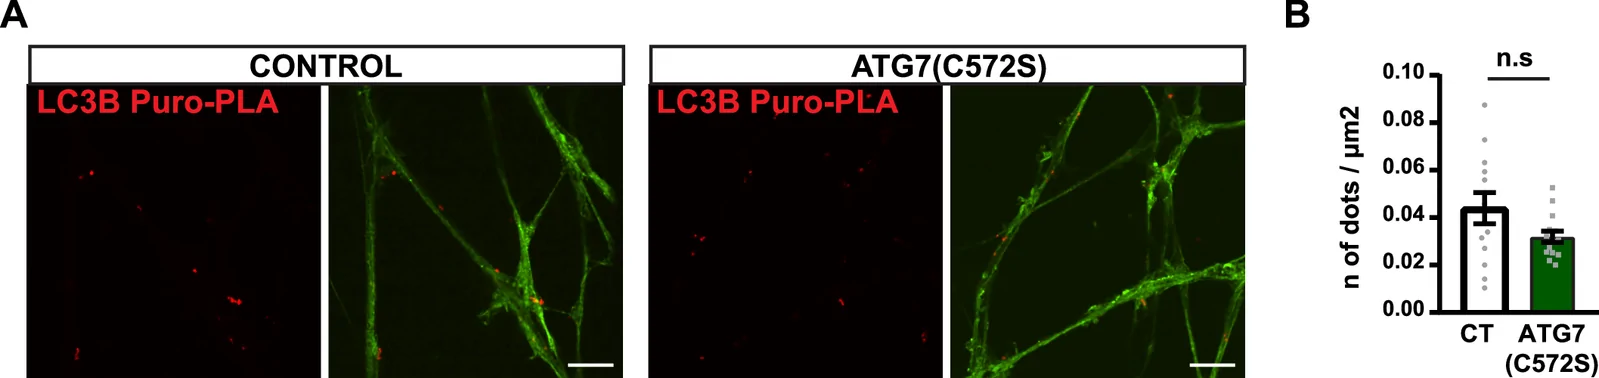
Expression of the ATG7(C572S) mutant does not affect LC3B synthesis in axons. (A) Images showing LC3B Puro-PLA signal in axons. Scale bar: 10 μm. (B) Quantification in the indicated conditions (CT, *n* = 13 fields of view; ATG7(C572S), *n* = 15 fields of view; *n* ≥6 explants, 3 exp). Mean ± SEM. (B) Unpaired *t* test.
